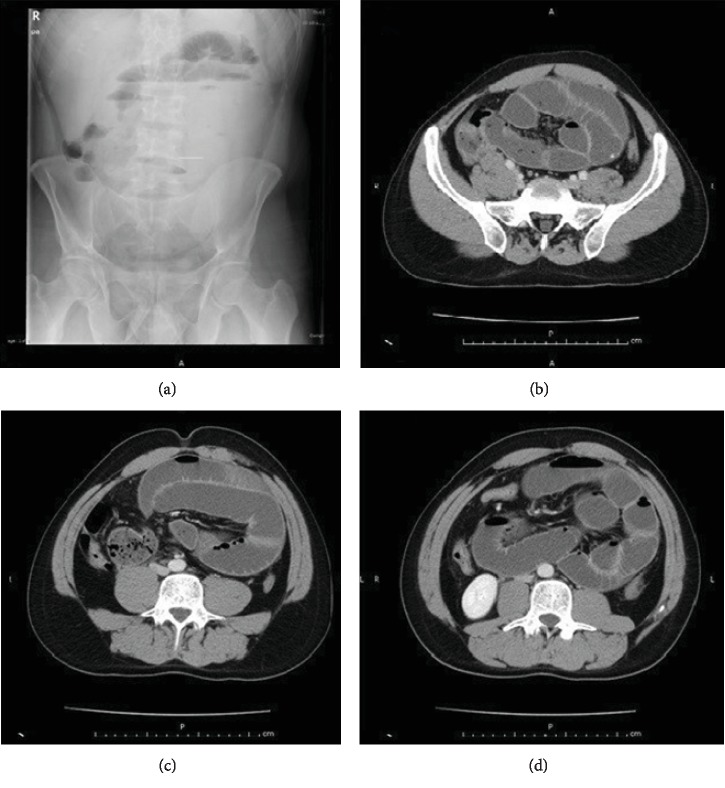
Case 1: air-fluid levels on X-ray showed an obstruction in the small intestine (a). Coronal sections of abdominal CT images indicated dilatation and thickening of the small intestine wall (b–d).
